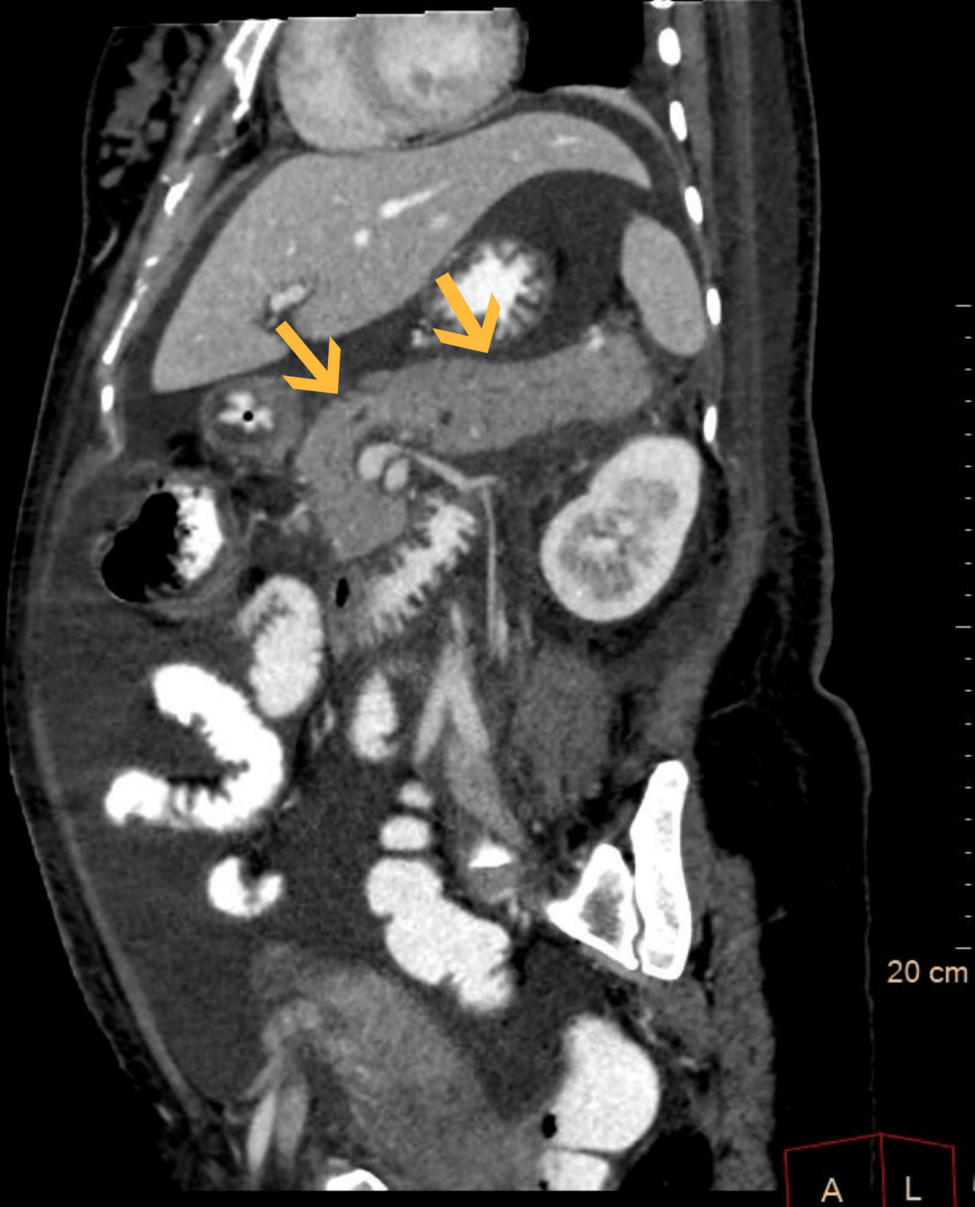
Sagittal sections of the contrast-enhanced computed tomography of the abdomen and pelvis The arrow marks indicate a non-enhancing pancreas in sagittal sections of the contrast-enhanced computed tomography of the abdomen and pelvis.
